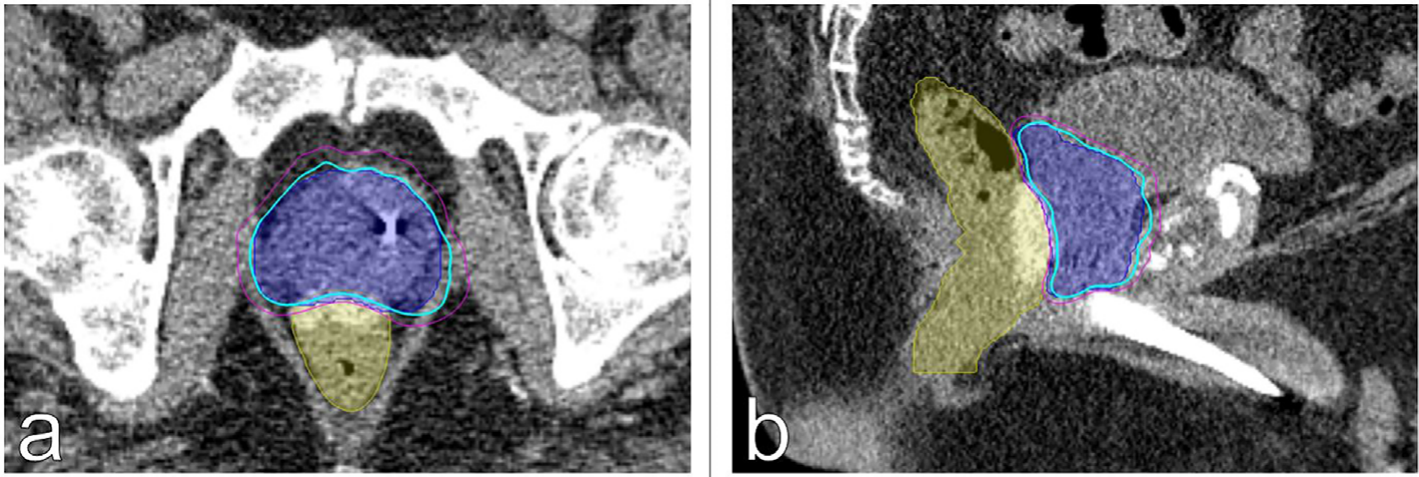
Treatment planning (A) axial and (B) sagittal computed tomography urethrogram images demonstrating the PTV (blue) and rectum with SpaceOAR Vue™ (yellow) are shown. Isodose lines shown as follows: 83% (blue), 75% (magenta).
